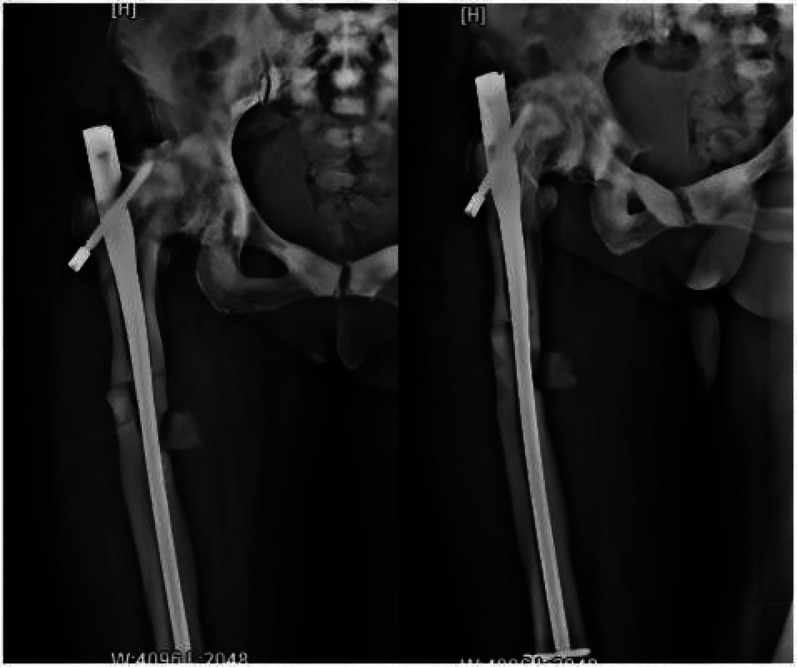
Postoperative x-ray images taken on the first day show that the intramedullary nail device is securely in place, and bone fragments are visible at the fracture site.
